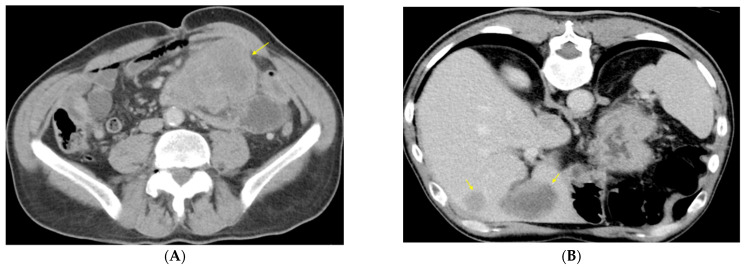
Abdominal CT scan at the first diagnosis, with i.v. administration of contrast material (venous phase shown): (A) large heterogeneous, hypoenhancing mass with infiltrative growth located in the left flank near jejunal loops and descending colon; (B) liver nodules/metastases hypoattenuating on unenhanced CT (not shown), enhancing at their periphery but less than surrounding liver parenchyma following contrast administration (A-yellow arrows).
